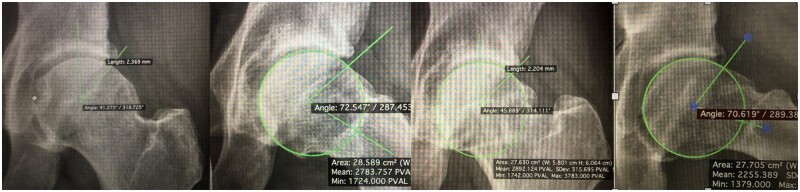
X-Ray of a patient who was treated using ChondroFiller gel (AP and lateral view).
